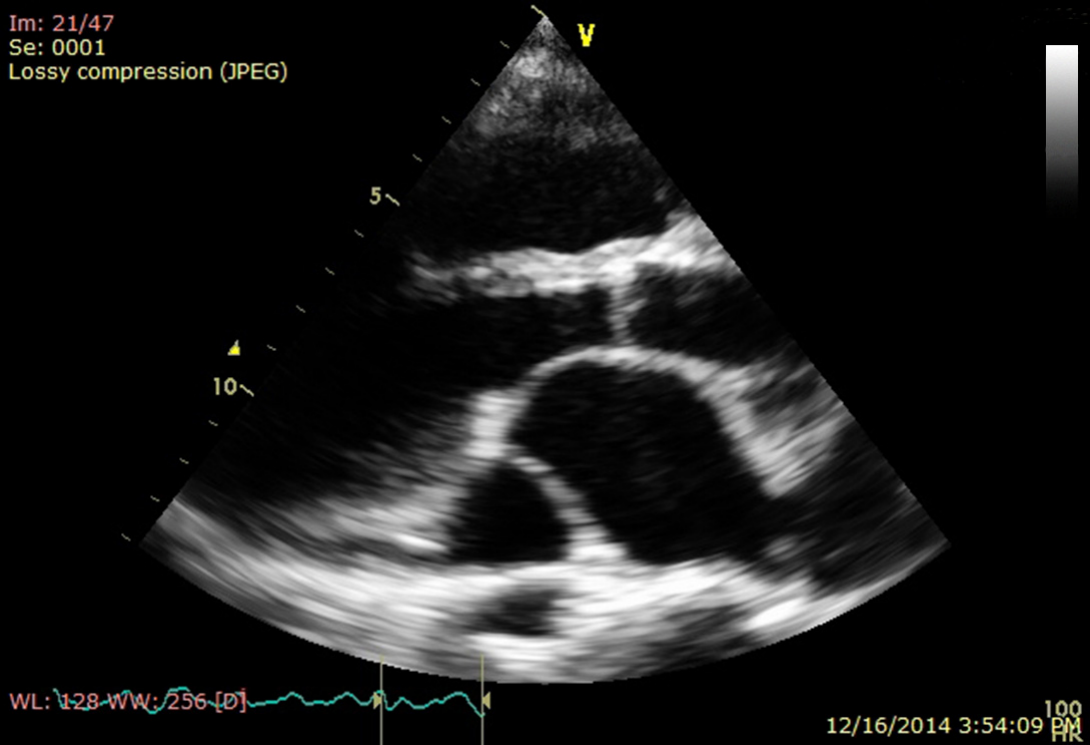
Transthoracic echocardiography, parasternal long axis view, showing dilatation of coronary sinus.
